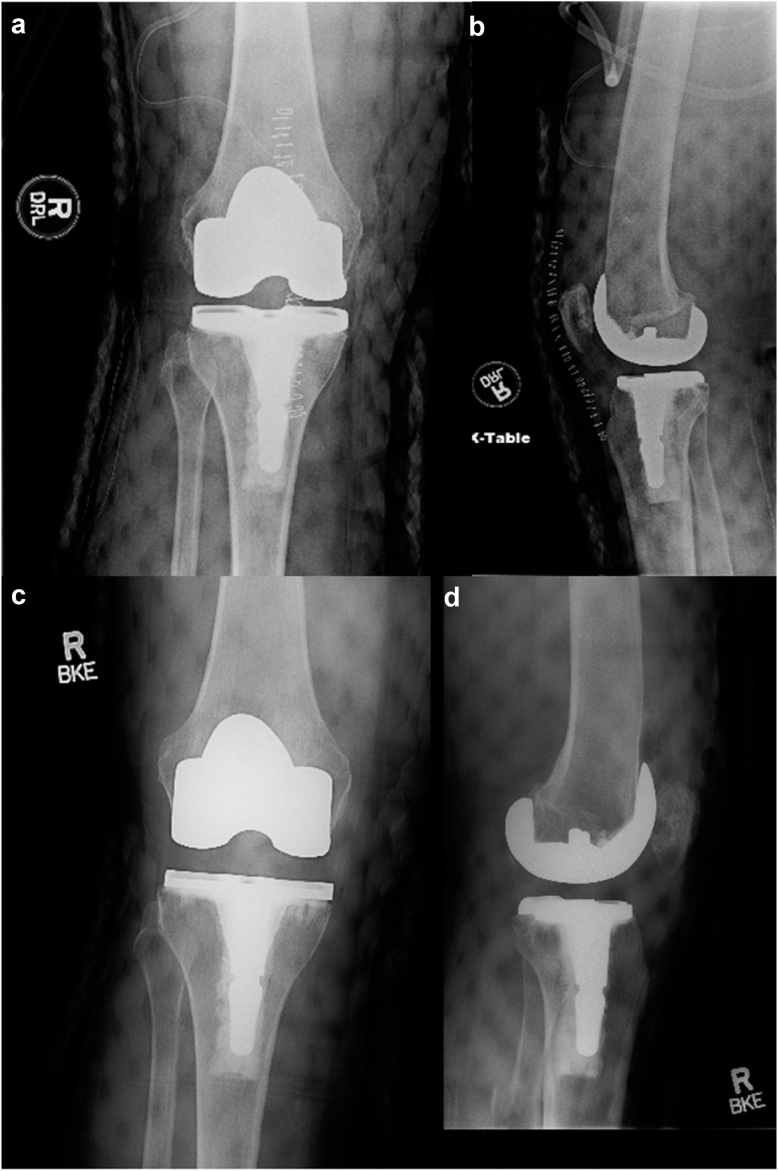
Demonstration of paradoxical instability. (a and b) Preoperative anteroposterior and lateral radiographs of a 72-year-old female with a Zimmer Biomet Persona posterior-stabilized total knee system, showing mid-flexion instability and flexion contracture, referred to as paradoxical instability. (c and d) Postoperative anteroposterior and lateral radiographs of the same patient. Intraoperatively, the components were confirmed to be well-fixed, and the polyethylene insert thickness was increased from a 12-mm cruciate-sacrificing insert to a 16-mm cruciate-sacrificing insert. This modification resulted in balanced flexion and extension gaps. Two years postoperatively, the patient is pain-free with a ROM from 0° to 125°.
